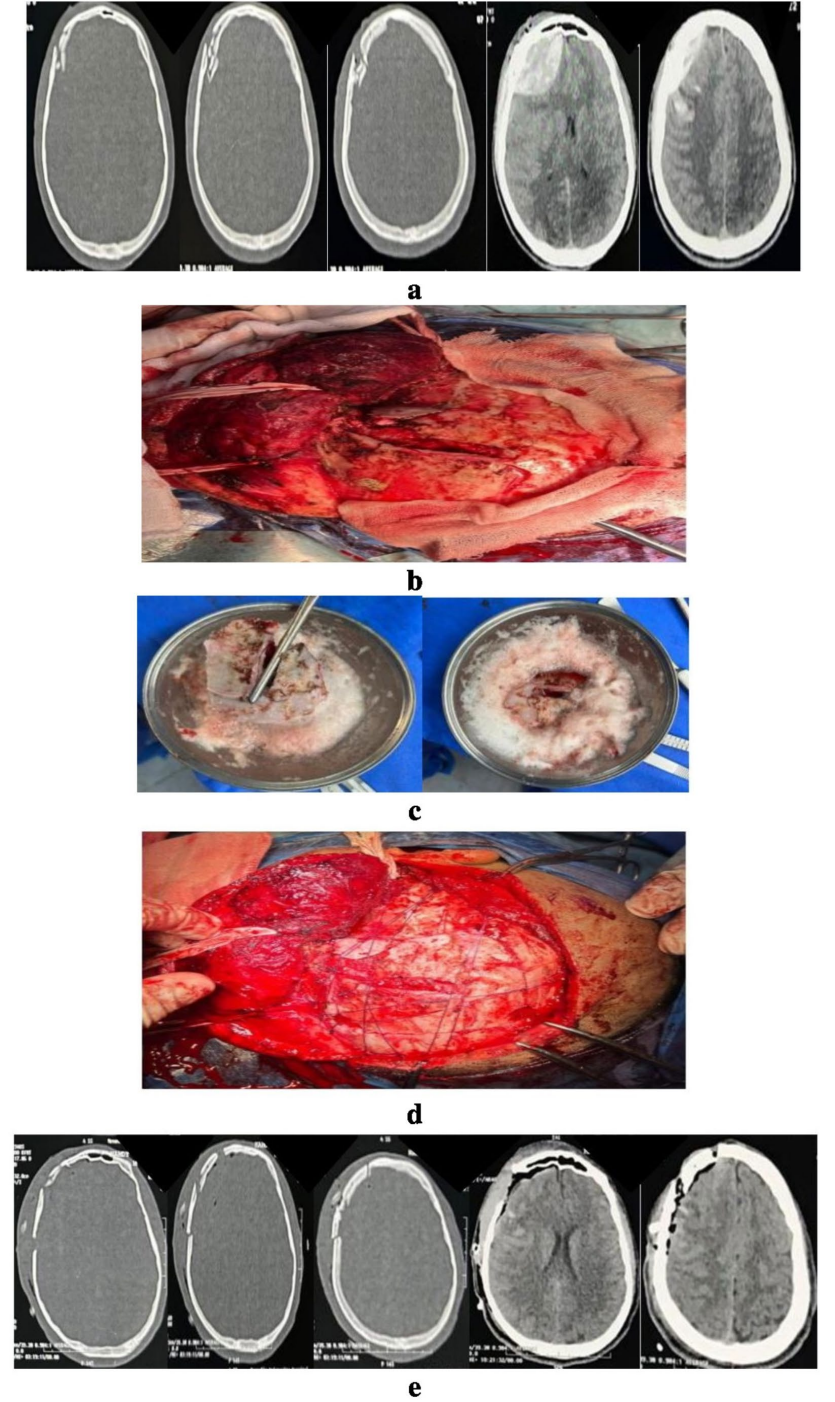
Young adult male patient arrived to our ER after RTA with GCS 12/15. (a) Preoperative CT scans showing right frontal DSF with underling EDH. (b) Intraoperative image showing the skull defect and depressed bone fragments. (c) Intraoperative image showing depressed bone fragments while being washed of in diluted hydrogen peroxide. (d) Intraoperative image showing defect reconstruction using autologous bone fragments fixed with a net of vicryl. (e) Postoperative CT scans showing good reconstruction of the skull defect
